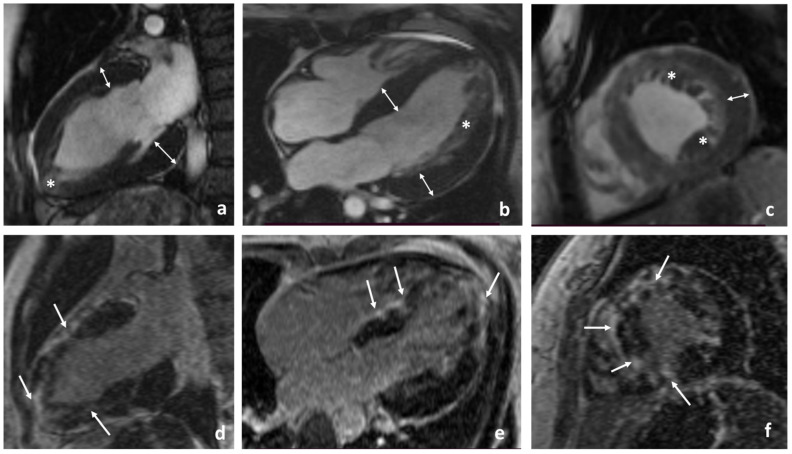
Cardiac MRI of the proband’s sister (III-3). (a–c) Cine mode, SSFP sequence; (a) long-axis two-chamber images, (b) long-axis four-chamber images, and (c) short-axis images. Asterisks mark the noncompacted layer in the apical region and throughout the walls of LV; arrows show the total hypertrophy of all the LV walls. (d–f) Delayed contrast enhancement; IR sequence with suppression of the signal from the myocardium. Arrows show extended intramyocardial fibrosis of a non-coronary origin in the LV apex, interventricular septum, and anterior and lateral LV walls.
